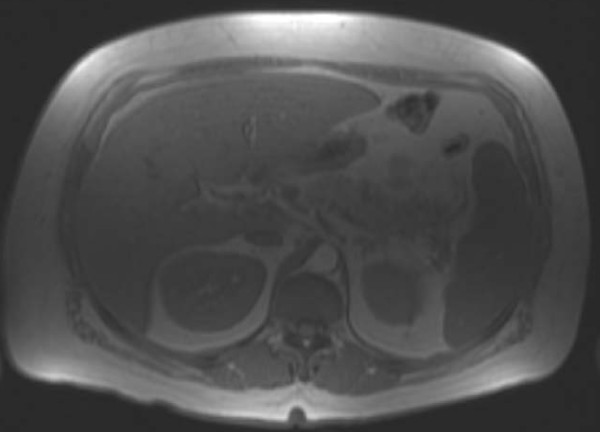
Magnetic resonance imaging scan of the liver with contrast enhancement obtained during the first week of admission showing a normal hepatobiliary tree.
